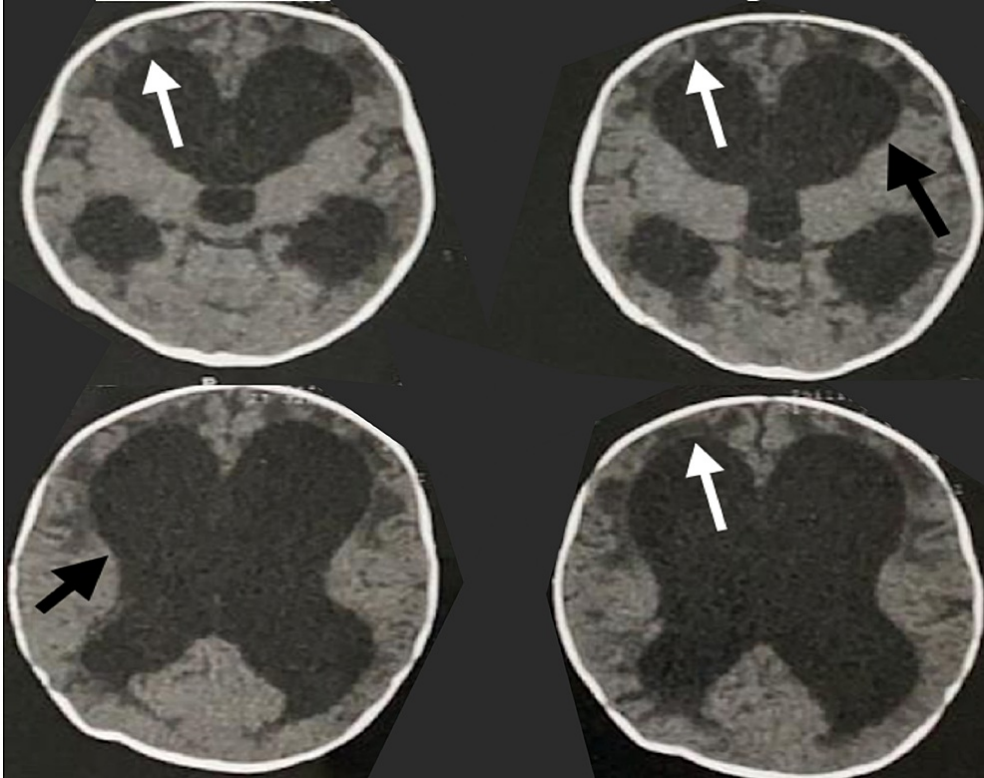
Axial computed tomography (CT) scan Shows enlargement of the ventricles (black arrows) with transependymal edema (white arrows).
